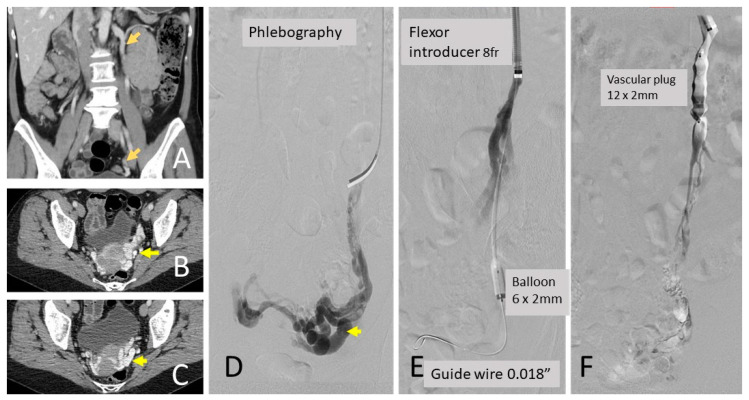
Pelvic varices. CT angiography: (A) coronal view shows the large caliber left ovarian vein (arrows), (B) axial section with left gonadal varices, (C) axial section of peri uterine varices, (D) phlebography of left ovarian vein with contralateral reflux, (E) phlebography with flexor-type 8Fr catheter, 6 mm × 2 mm balloon occluding the ovarian vein on a 0.018′ microguide. Injection of distal ethoxysclerol; (F) ovarian vein occlusion with proximal plug.
